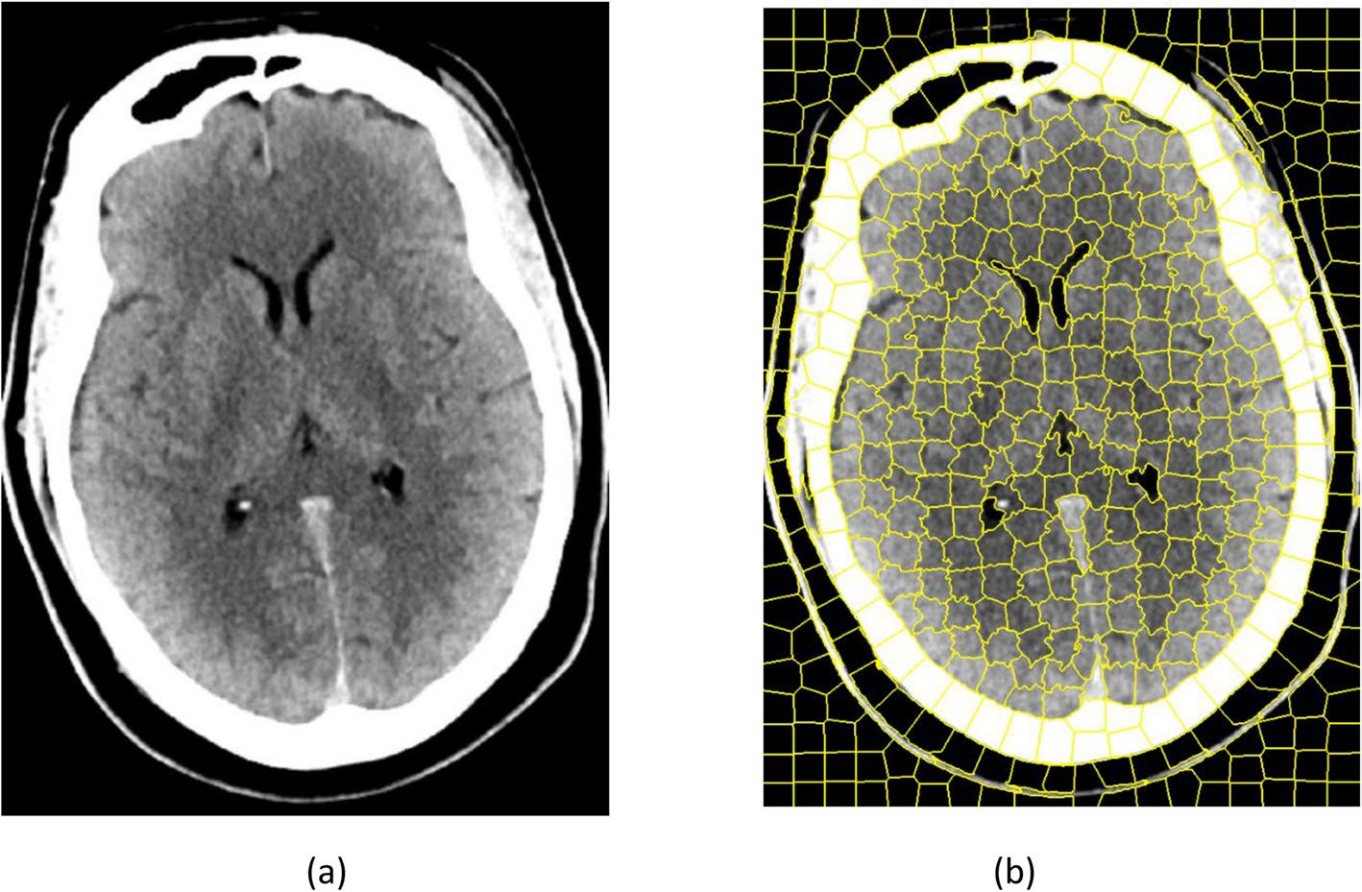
a Brain CT; b Segmented white matter and gray matter
